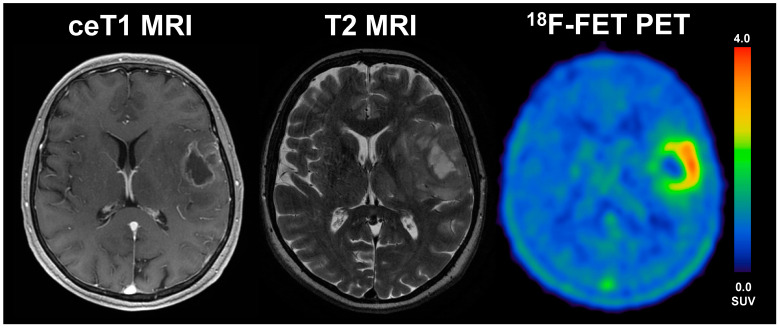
57-year old female with glioblastoma (WHO grade IV, IDH wildtype, *MGMT* unmethylated) and an overall survival of 4.9 months (STS). The diameter of CE on T1 MRI was 31 mm, the volume of CE was 6 mL, the BTV in FET-PET was 39.1 mL, the TTP_min_ was 12.5 min and TBR_max_ was 3.9. The patient underwent radiochemotherapy.
